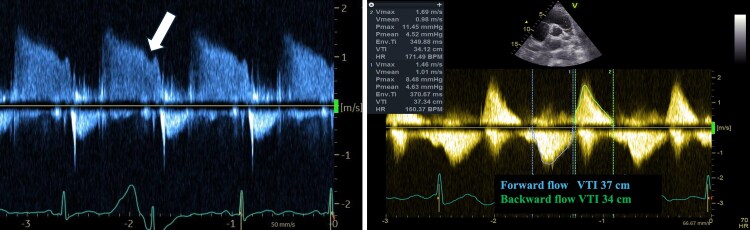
Continuous-wave Doppler signal in pulmonary regurgitation. Left panel shows a typical CWD signal in a patient with mild PR, with high early velocity following valve, a slow deceleration due to decreasing PA–RV gradient during diastole until the lower end-diastolic velocity is reached with fast pressure gradient drop towards pressure equalization. The white arrow indicates the A-dip, i.e. a fast decline in velocity due to atrial kick. Note also that in mild PR, the VTI of the PR signal is much larger than the forward signal. In the right panel, a severe PR CWD signal is shown. The CWD signal in severe PR is smaller and intense (almost equal pixel intensity compared to the forward signal) and with a fast deceleration slope, resulting in a small backward VTI of 34 cm (green tracing), almost similar to the forward VTI (blue tracing), indicating very large EROA.
